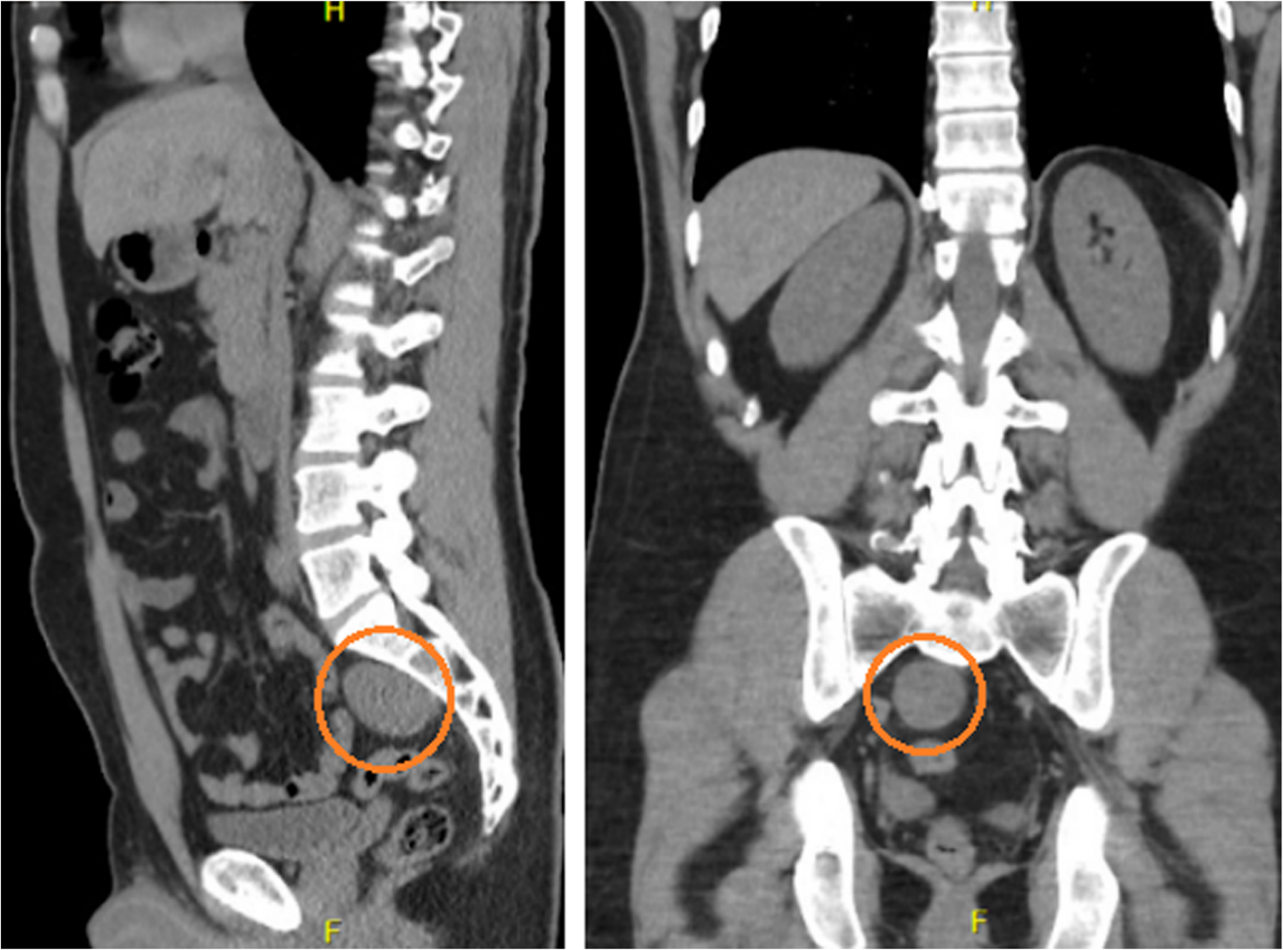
CT of the sagittal and coronal planes of the presacral region, respectively. Note the schwannoma (red circle), with dimensions of 4.4 × 3.9 × 3.4 cm
